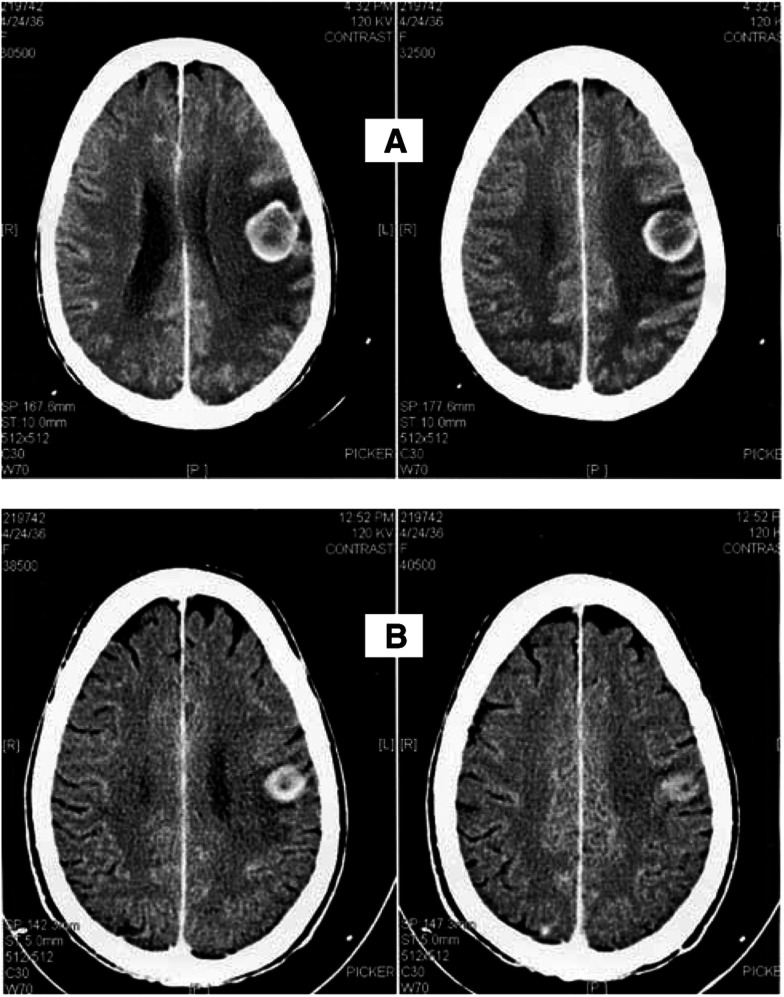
Case 4: Brain CT-scan at baseline (A) and after 3 months of ZD1839 therapy (B). Brain metastasis from NSCLC responding to ZD 1839 therapy. This patient has been pretreated with three lines of chemotherapy including platinum and taxanes, and received ZD 1839 after whole-brain radiotherapy failure.
